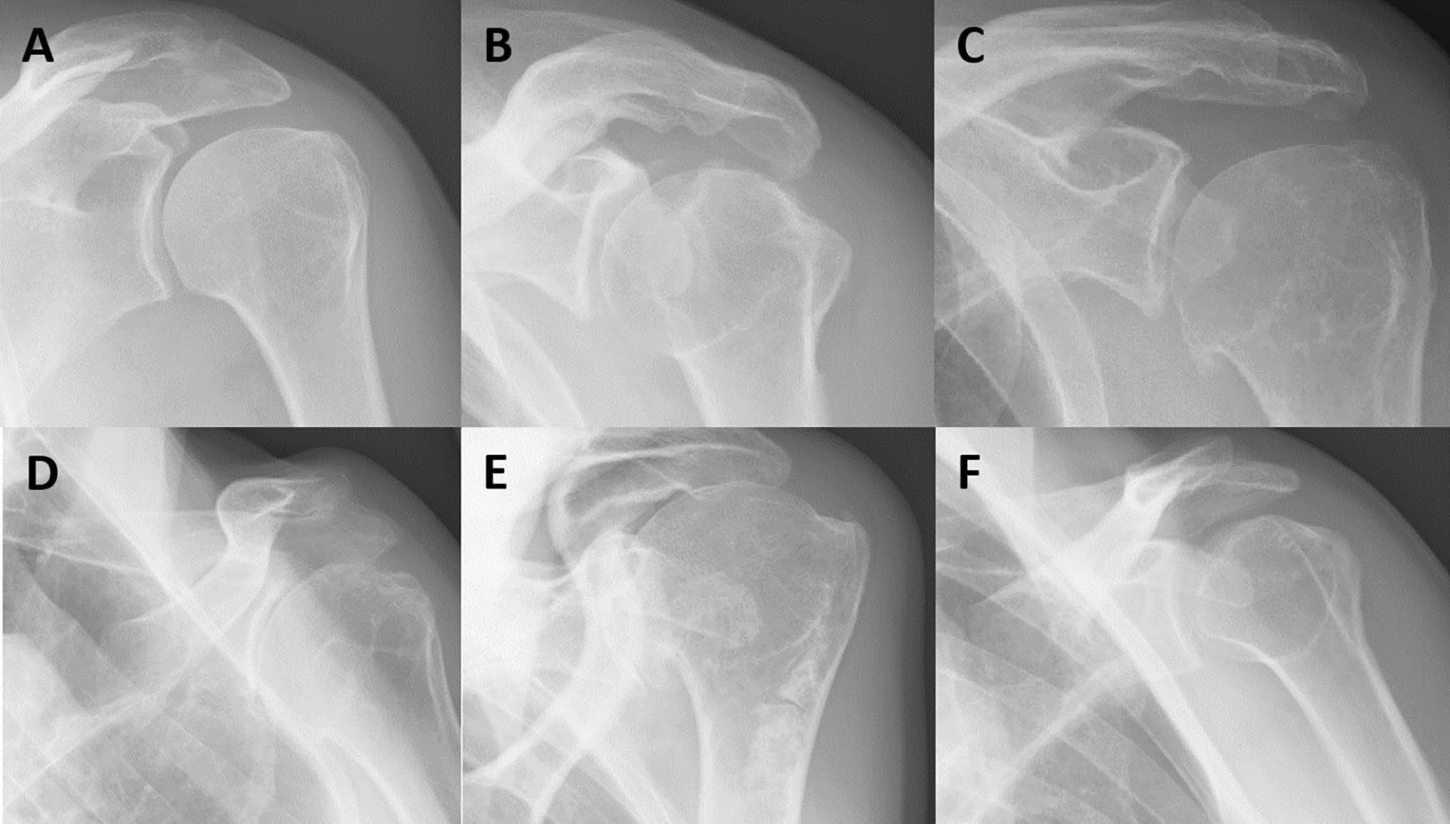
Radiographic glenohumeral OA in patients with controlled acromegaly according to a modified Kellgren and Lawrence scoring method. Examples of the different scores of the modified Kellgren and Lawrence (KL) scoring system of the glenohumeral joint in our acromegaly patients, based on previously described definitions [49–53]. A KL score 0, B KL score 1, C KL score 2, D KL score 3, and E KL score 4. F Significant joint space widening (JSW), characteristic for acromegalic arthropathy
